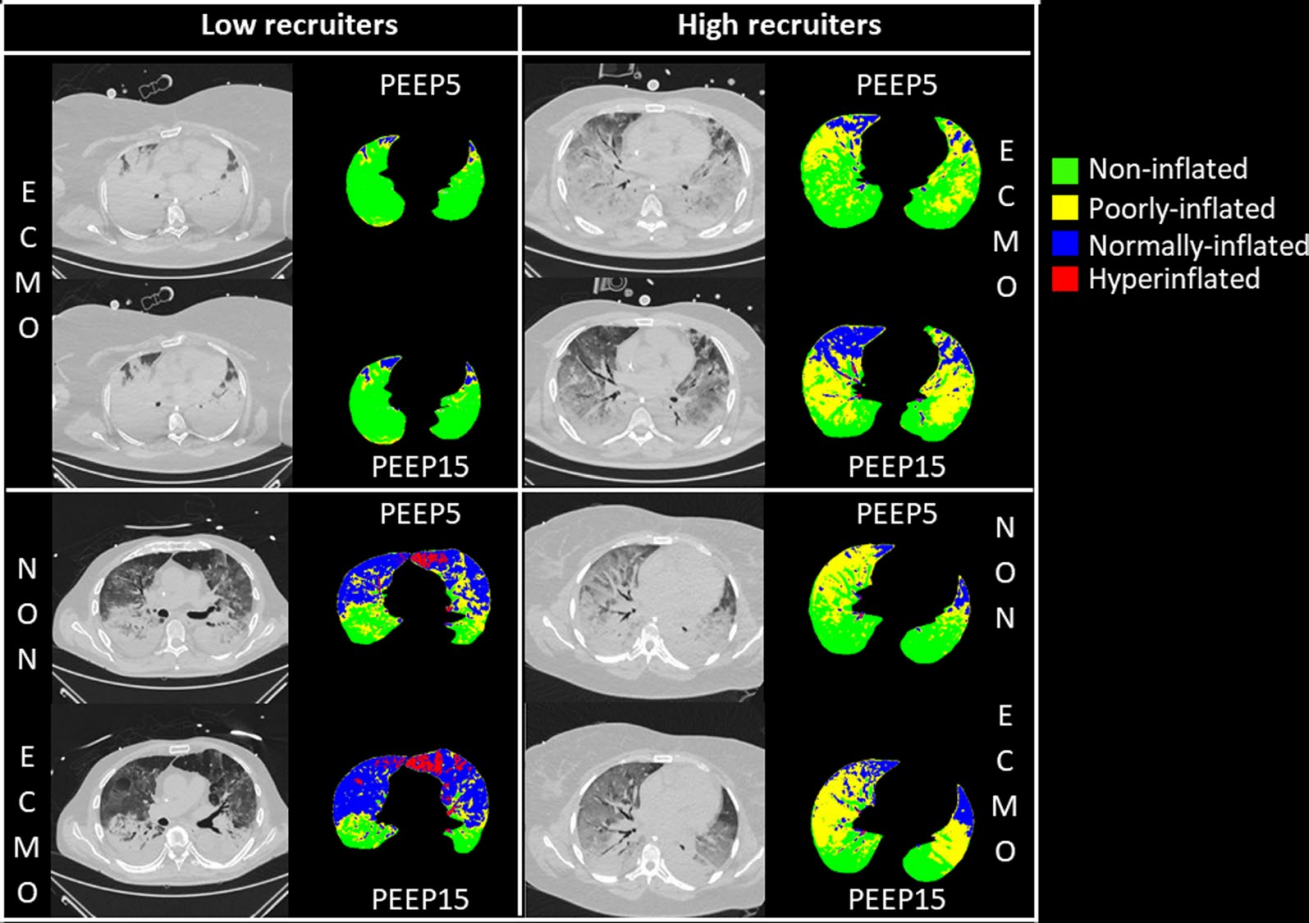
CT images acquired at the mid-chest level in 4 representative patients. ECMO patients are presented in the uppermost quadrants, while non-ECMO patients are presented in the lowermost quadrants. Patients with ∆PEEP_5–15_-induced recruitment below the median value of the whole population (deemed as low recruiters) are presented in the leftmost quadrants, while patients with PEEP_5–15_-induced recruitment above the median value of the whole population (deemed as high recruiters) are presented in the rightmost quadrants. In each quadrant, the upper two images were acquired at PEEP 5 cmH_2_O (one raw CT image on the left and one quantitative parametric CT on the right), and the lower two were acquired at PEEP 15 cmH_2_O. The color code used for parametric images is provided in the figure legend. CT, computed tomography, ∆PEEP_5–15_-induced recruitment, amount of recruitable lung between PEEP 5 and 15 cmH_2_O, ECMO, extracorporeal membrane oxygenation, PEEP, positive end-expiratory pressure
